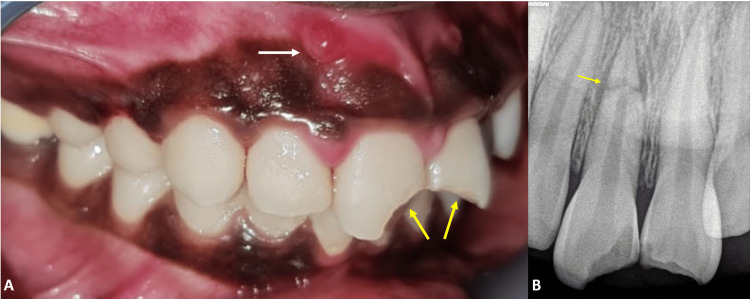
(A) Pre-operative clinical image showing soft tissue swelling at the apical region of 11 as represented by the white arrow, fracture lines at the coronal portion of 11 and 21 as represented by the yellow arrows. (B) Pre-operative radiograph with the yellow arrow showing HRF at the junction of the middle and apical third of tooth 11. HRF: horizontal root fracture.
